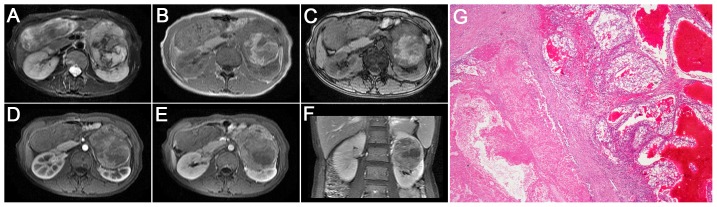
Translocation RCC in a 29-year-old woman (patient 5 in Table 1–2) with a left palpable abdominal mass. A–C, Axial T2WI and plain (non-contrast) T1WI (B, IP in phase; C, OP out of phase) showing a large, well-defined, irregular mass (T2, high-low heterogeneous signal intensity; T1, iso-signal intensity) with a large patchy hemorrhage and necrosis in the center of the mass. D–F, CMP and NP gradient-echo MR images showing heterogeneous and prolonged enhancement of the mass. G, The tumor cells are polygonal, with voluminous eosinophilic cytoplasm containing a few hyaline nodules and forming a papillary structure (HE 10 & 10).
